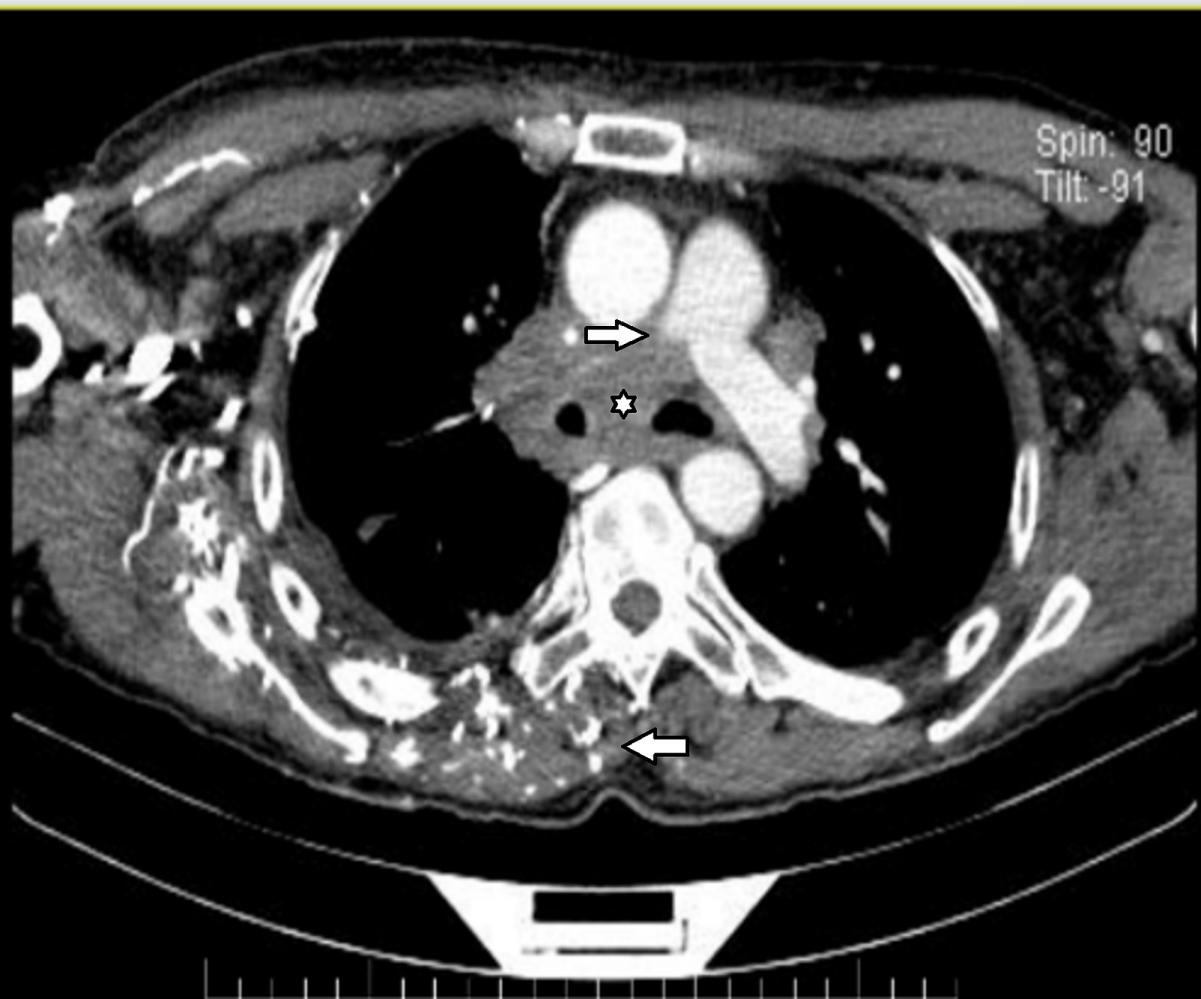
Mediastinum computed tomography. Arterial phase. Chest adenopathy in the right mediastinum that conditions displacement of the larynx and esophagus as well as compression of the brachiocephalic trunk (star) and right pulmonary artery with obstruction of more than 90% (top arrow) with collateral circulation (bottom arrow).
